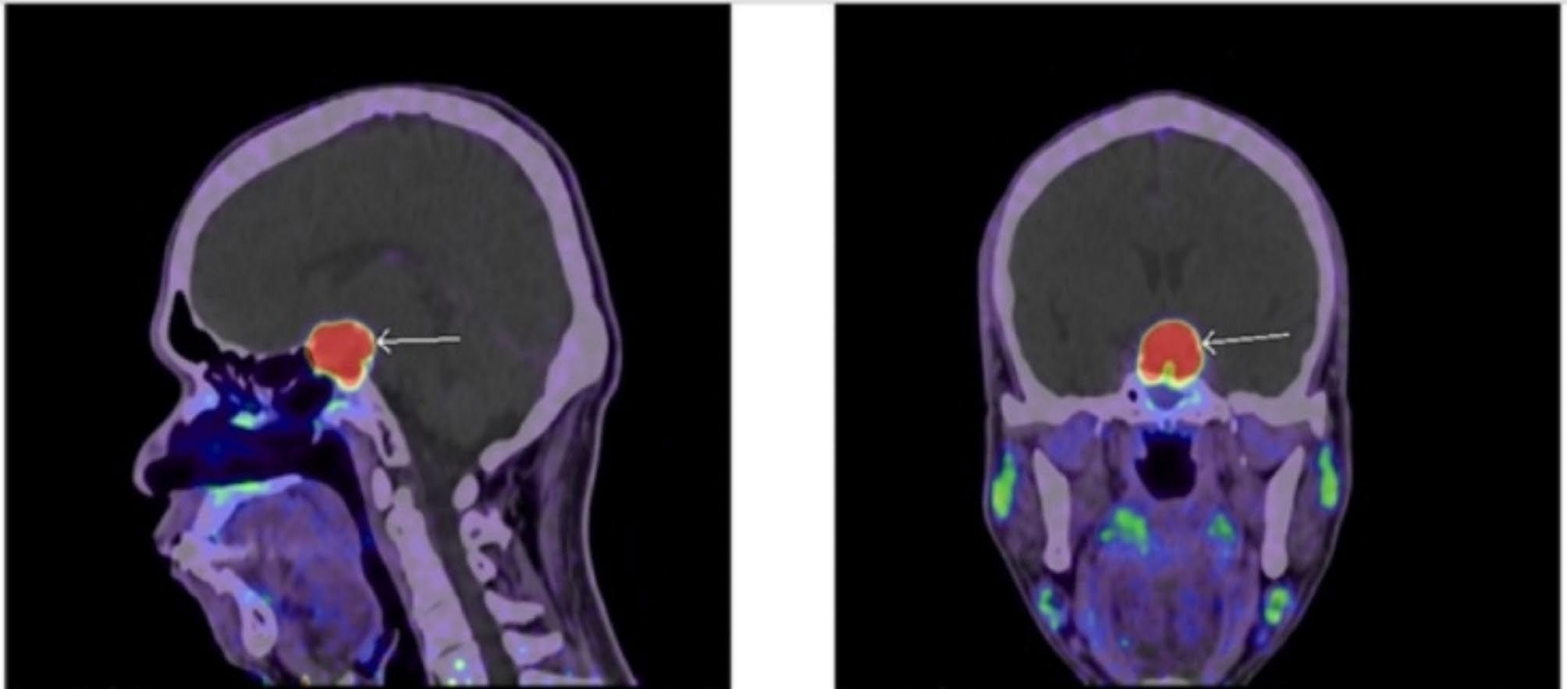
Sagittal and coronal views of 68-Gallium DOTA peptide Positron Emission Tomography (Ga68 DOTATATE PET CT) of the pituitary gland with the arrow showing significant uptake corresponding to pituitary macroadenoma
